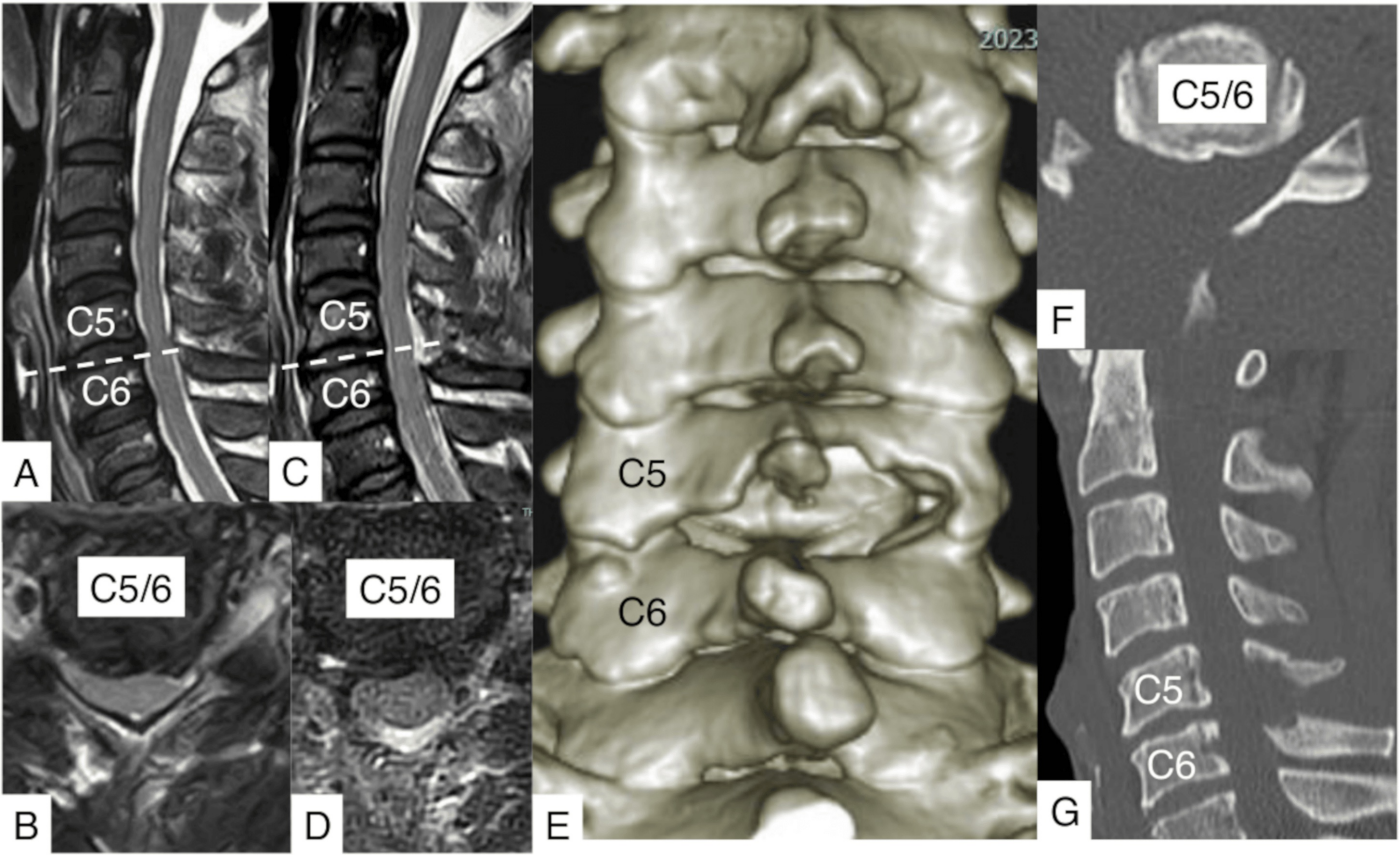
Pre- and postoperative radiological findings of Case 1. A, B: Preoperative magnetic resonance images (MRI) show C5/6 spinal cord compression and right foraminal stenosis because of disc herniation (A: sagittal image, B: axial image). C, D: Postoperatively, the spinal cord and right C6 nerve root were decompressed (C: sagittal image, D: axial image). E: Postoperative 3-dimensional reconstructed computed tomography (CT) and plain CT images show central decompression and foraminotomy at C5/C6. F, G: Postoperative CT images revealed posterior decompression at C5/C6 (F: axial image, G: sagittal image).
